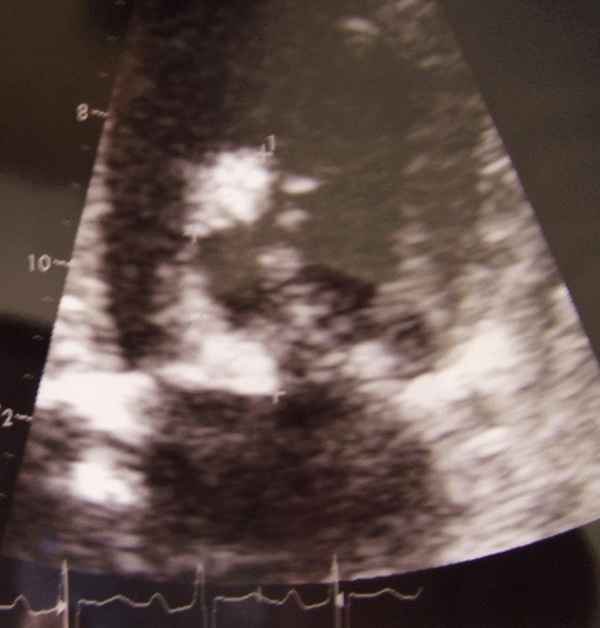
Pre-operative transthoracic echocardiography showing mitral vegetation and mitral chordal rupture.
